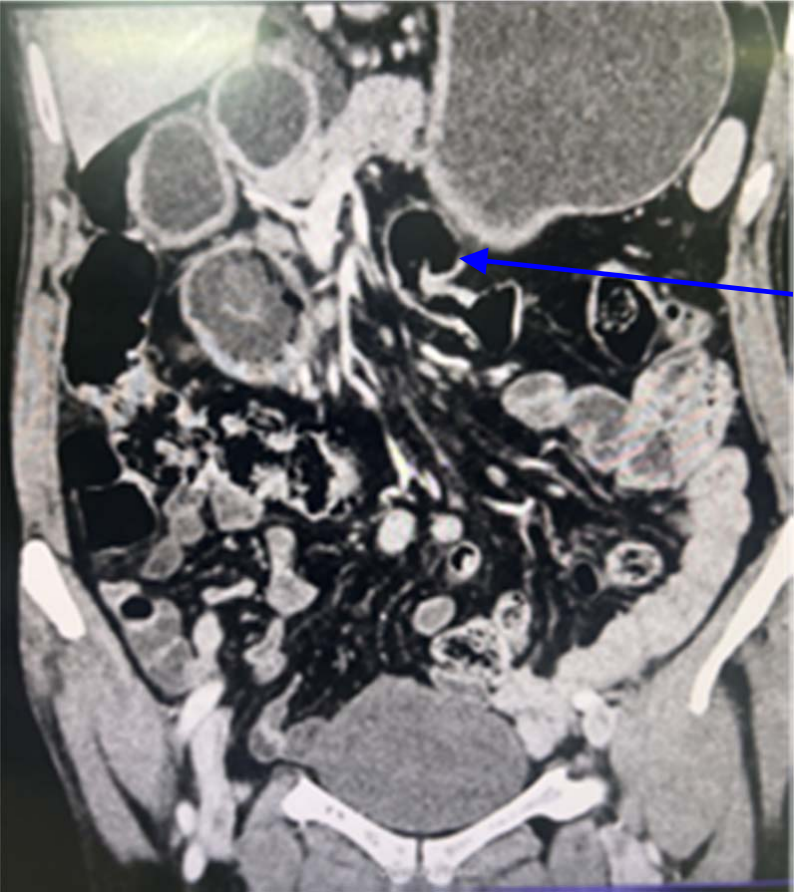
Computed tomography scan coronal section with arrow showing narrowing at duodenojejunal flexure.
